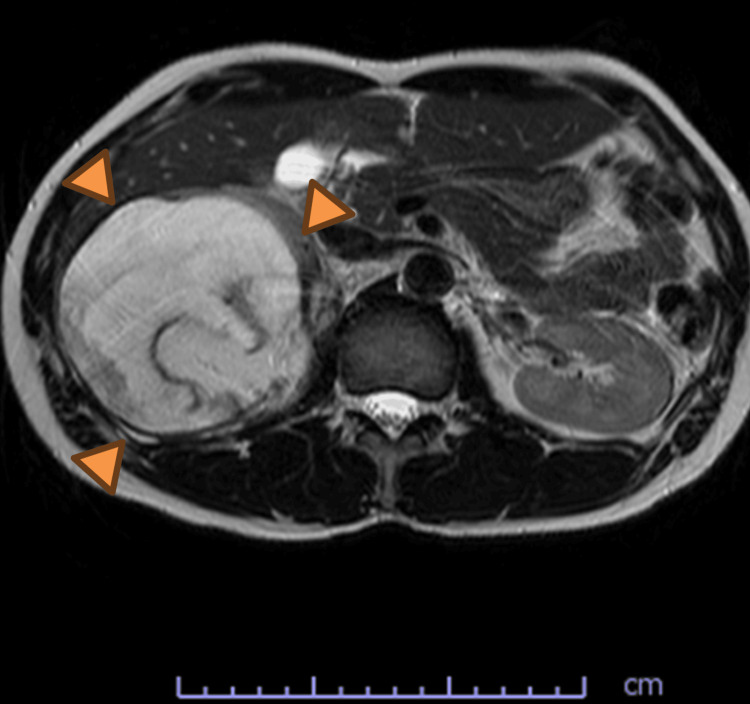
Abdominal MRI (T2-weighted image) findings MRI demonstrates a heterogeneous mass with internal septations and fat components MRI: magnetic resonance imaging
